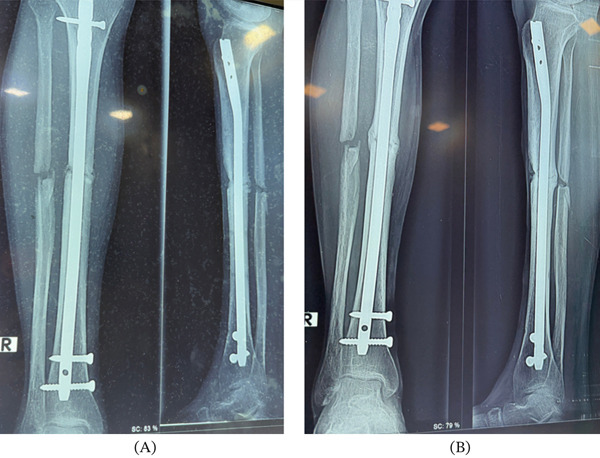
Late healing and final outcome. (A) Anteroposterior and lateral radiographs dated June 13, 2025, showing bridging callus formation and advanced healing. (B) Anteroposterior and lateral radiographs dated March 27, 2026, confirming solid union with cortical continuity and remodeling.
